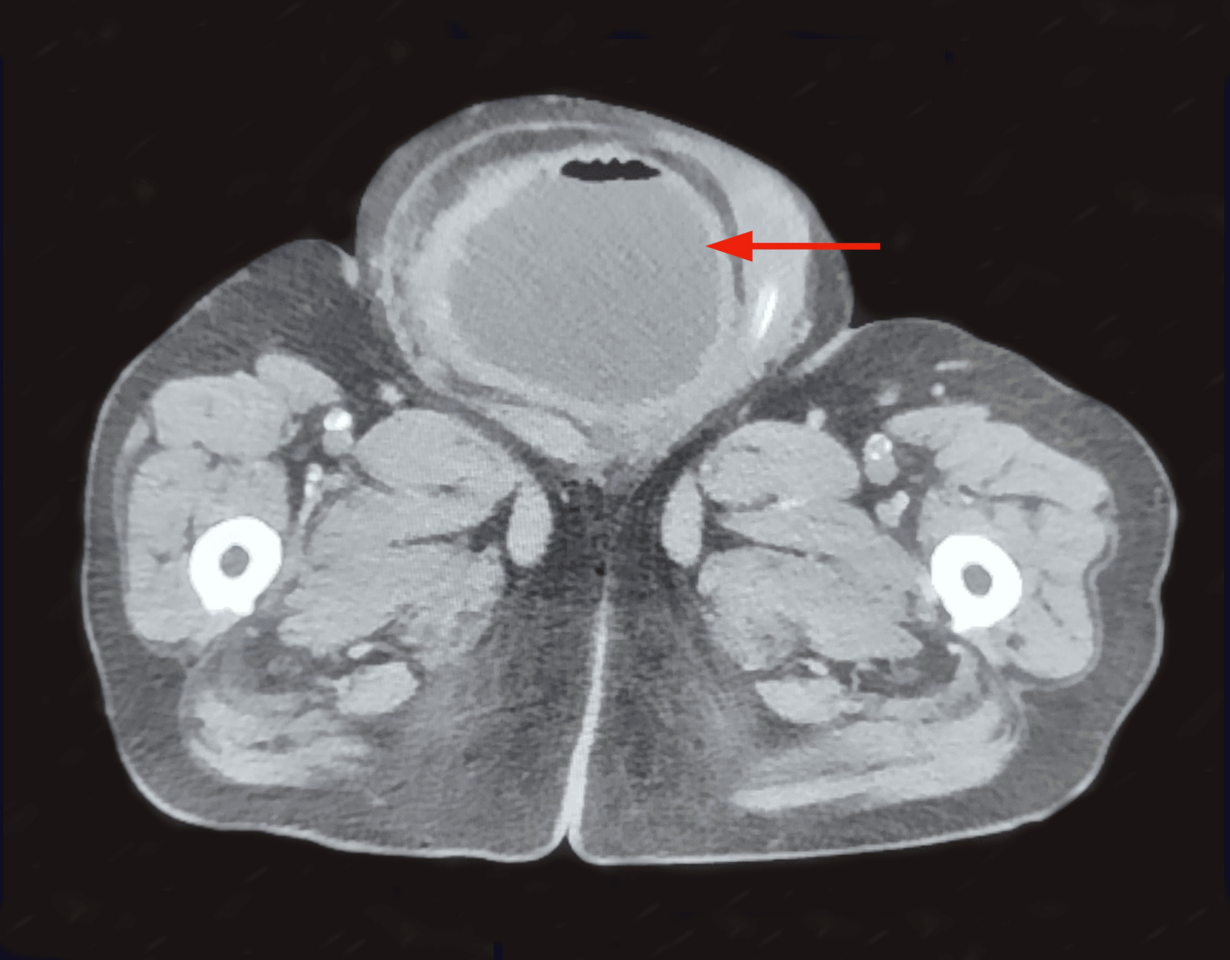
Transverse section of an abdominopelvic CT scan demonstrating a bladder-containing inguinoscrotal hernia (red arrow).
